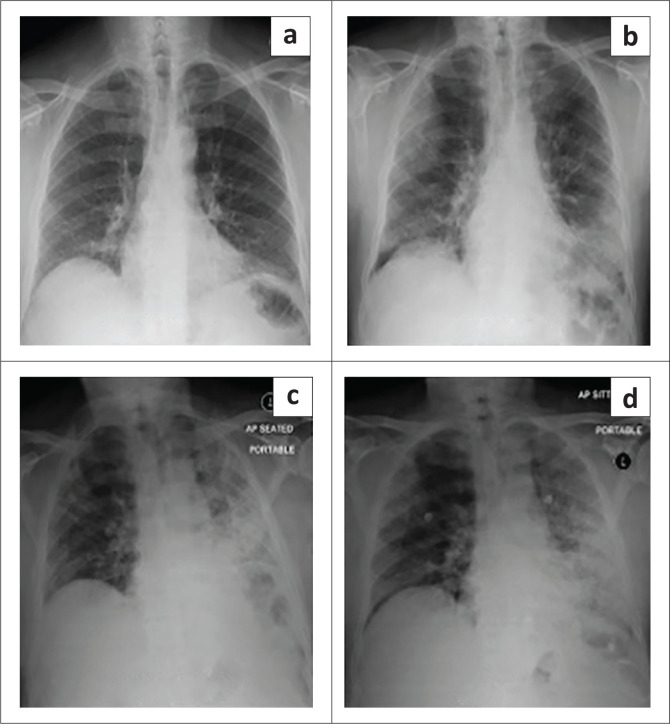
Chest radiographs from (a) 03 October 2013, (b) 27 June 2020, (c) 03 July 2020 and (d) 11 July 2020): 2013 normal baseline radiograph. Serial images demonstrate progressive unilateral left lower lobe and lingular air space opacification, consolidation and volume loss. Focal right upper lobe consolidation with ‘air bronchograms’.
